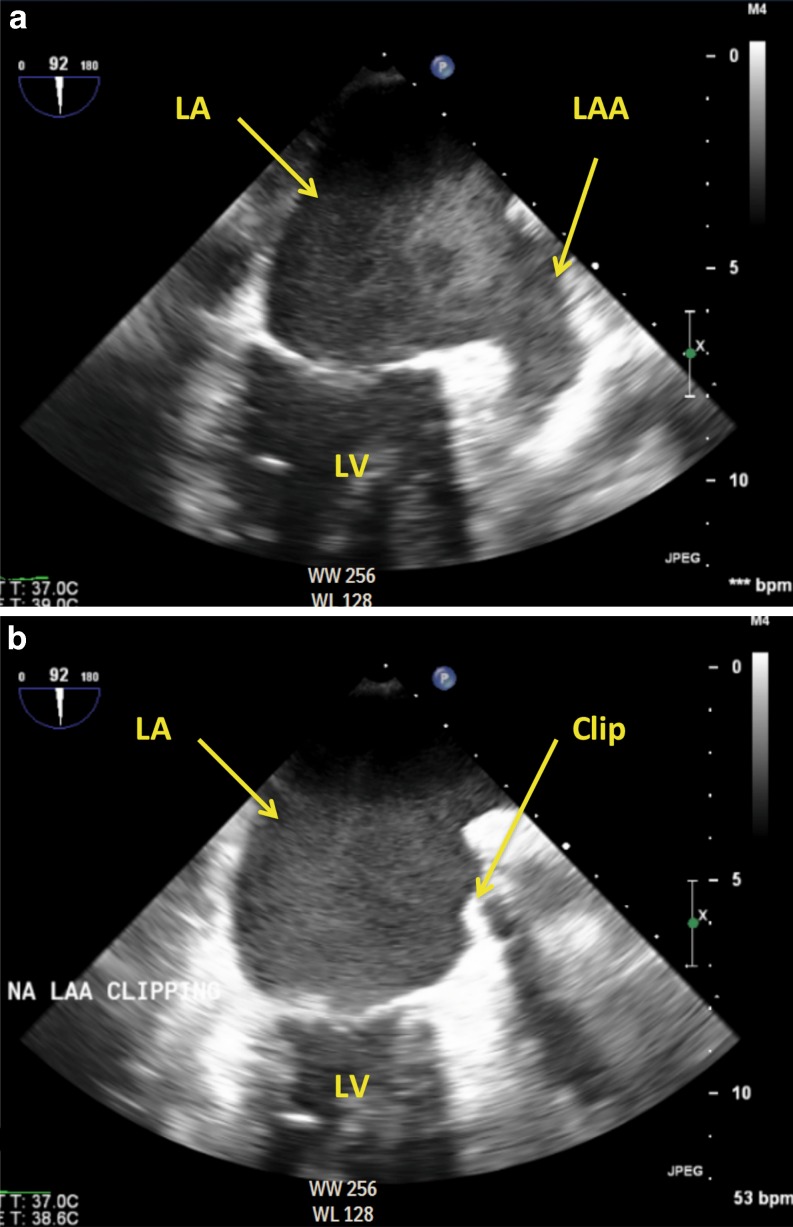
a Preoperative transoesophageal echocardiography demonstrating spontaneous contrast in the left atrial and left atrial appendage. b After placement of the Atriclip PRO device. Complete closure of the LAA is obtained. (*LA* left atrium, *LAA* left atrial appendage, *LV* left ventricle)
